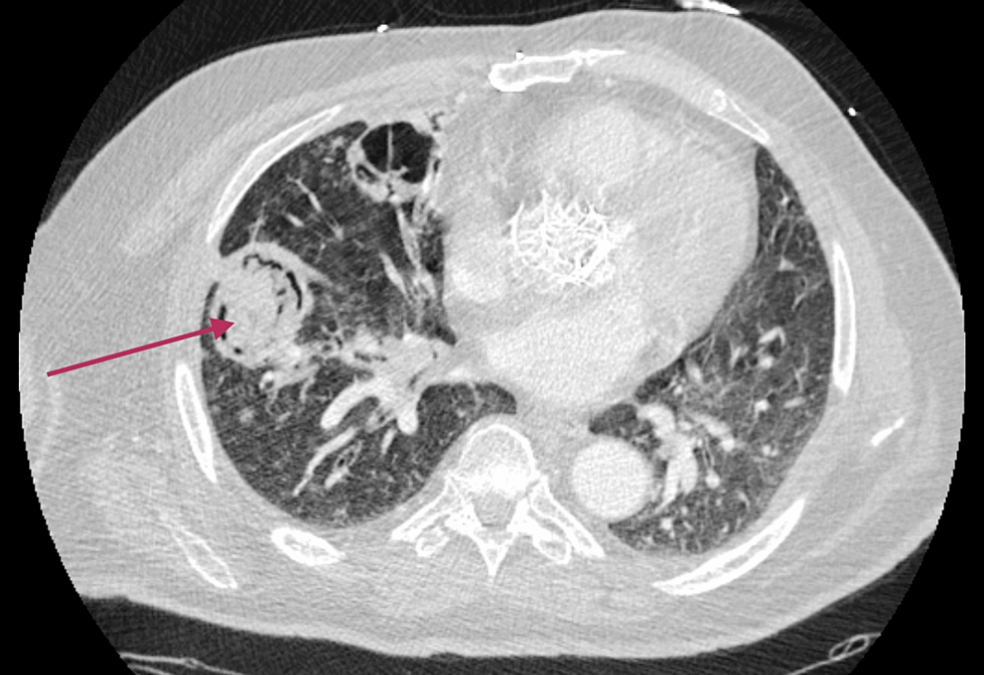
CT chest of a patient with the development of invasive pulmonary aspergillosis (IPA) and associated aspergilloma CT chest of a patient with the development of IPA as a sequela of post-COVID-19 pneumonia. Axial image showing a 4.6 x 4.2 cm cavitary lesion in the right lower lobe with intracavitary mass/debris representing an aspergilloma.
